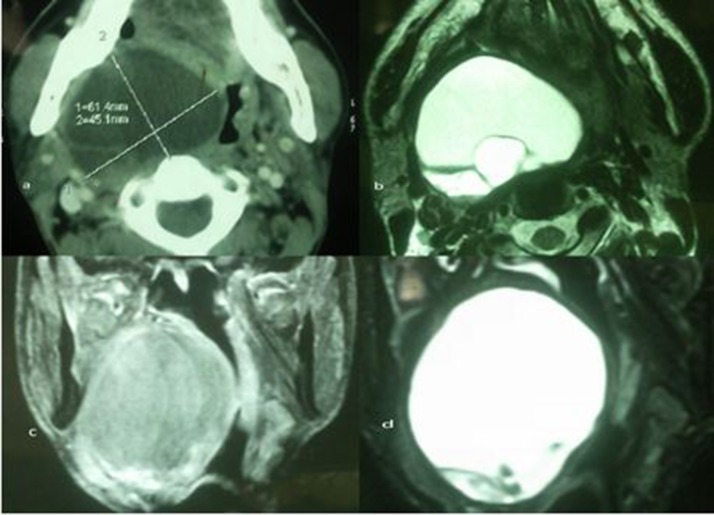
Computed tomography findings of the parapharyngeal ancient schwannoma: axial image showing a 6.1cm × 4.5cm mass in the right parapharyngeal space (top left), axial T2 weighted image showing the oropharyngeal mass with brightly hyperintense signal and few internal septa posteriorly (top right), coronal T2 weighted post-contrast image showing peripheral enhancement of solid components inferiorly (bottom left) and coronal T2 weighted image showing hyperintensity in the mass with septa and nodules seen inferiorly (bottom right)
